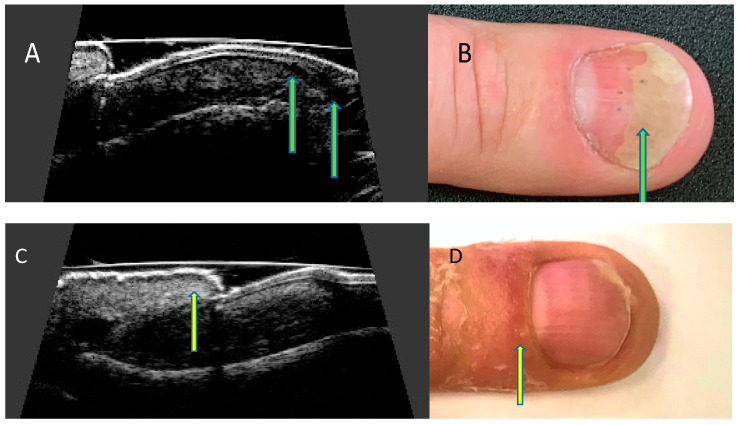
Ultrasonographic changes of the nail apparatus in psoriasis. Onycholysis. (A) Separation of the nail plate from the nail bed—ultrasound longitudinal view (arrows), (B) separation of the distal edge of the nail bed—nail (arrow). Inflammation of the posterior nail shaft. (C) Thickening of the nail shaft—ultrasound longitudinal view (arrow), (D) swelling of the nail shaft (arrow).
